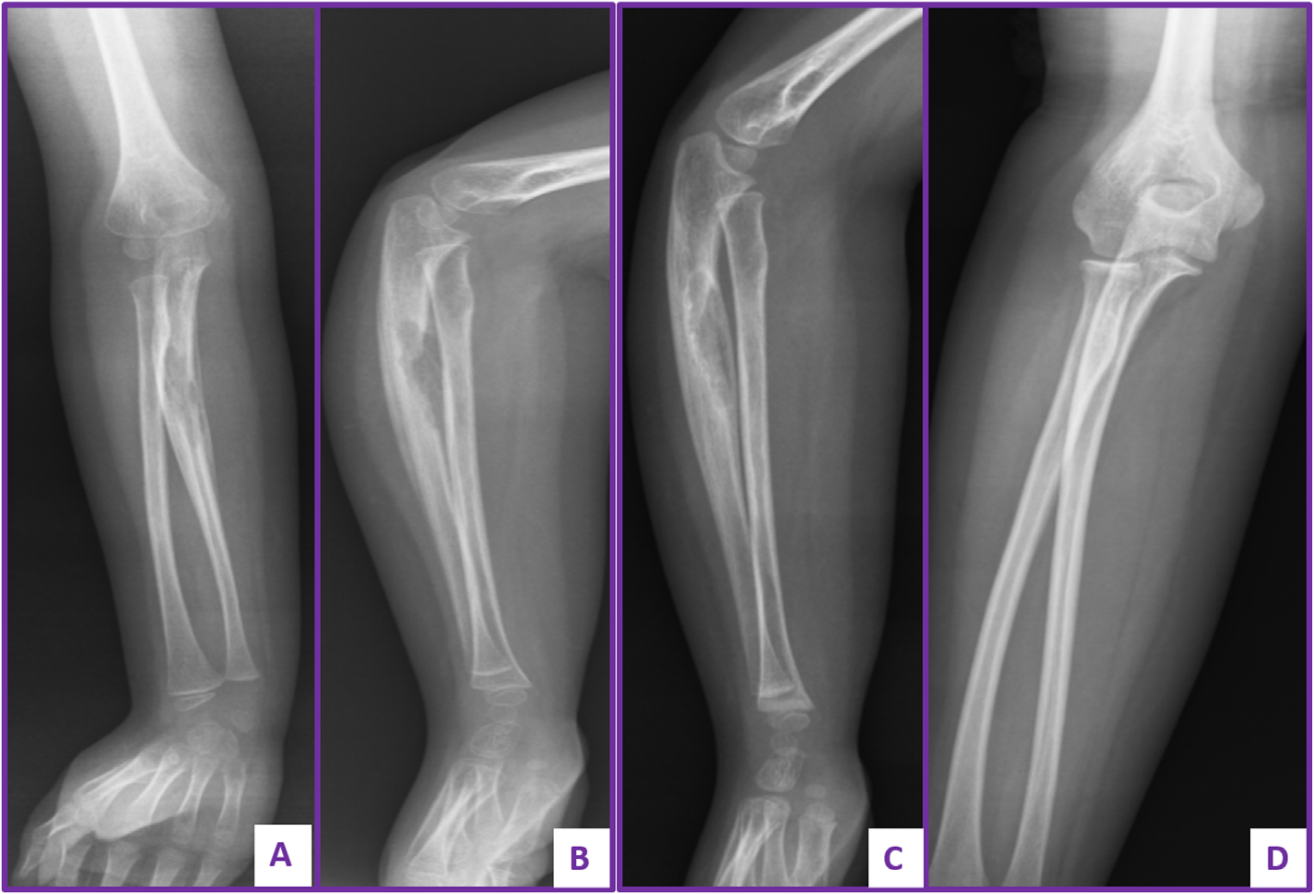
Plain radiograph of forearm of a 5-year-old girl diagnosed with LCH showing large osteolytic lesion of the ulna managed conservatively. (A and B) Anteroposterior and lateral views showing the large sized osteolytic lesion of the ulna. (C) Follow-up after two years showing partial remodeling. (D) Follow-up radiograph showing complete remodeling.
